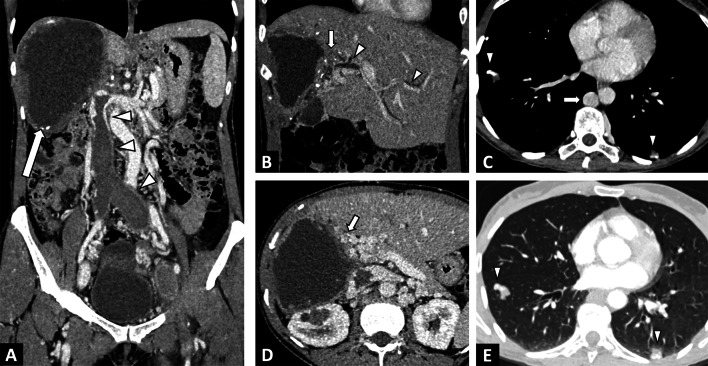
Alveolar echinococcosis lesion of the right liver in a 49-year-old woman discovered due to left leg edema. A CT image in the coronal plane at the portal phase showing alveolar echinococcosis of the right liver (arrow) associated with invasion of the inferior vena cava, responsible for thrombosis, extending to the common iliac veins (arrowheads). B CT image in the coronal plane obtained during the portal phase: infiltration to the pedicle and biliary convergence (arrows) and biliary dilatation of the left lobe (arrowheads). C CT image in the axial plane obtained during the portal phase showing portal cavernoma of the liver pedicle secondary to hilar alveolar echinococcosis infiltration. D Same examination, soft tissue window: calcified lung nodules (arrowheads). Hypertrophy of the azygos vein secondary to inferior vena cava thrombosis (arrow). E Same examination, lung window: calcified lung nodules (arrowheads)
